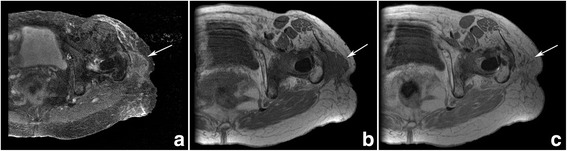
(a) Axial MR STIR images of the left hip at the level of the greater trochanter show an irregular subcutaneous mass (white arrow) involving the iliotibial band and abutting the greater trochanter. The mass is primarily hyperintense on fluid-sensitive sequences. On axial T1-weighted pre-gadolinium (b) the mass is isointense to muscle and enhances avidly following contrast administration on post-gadolinium images (c)
